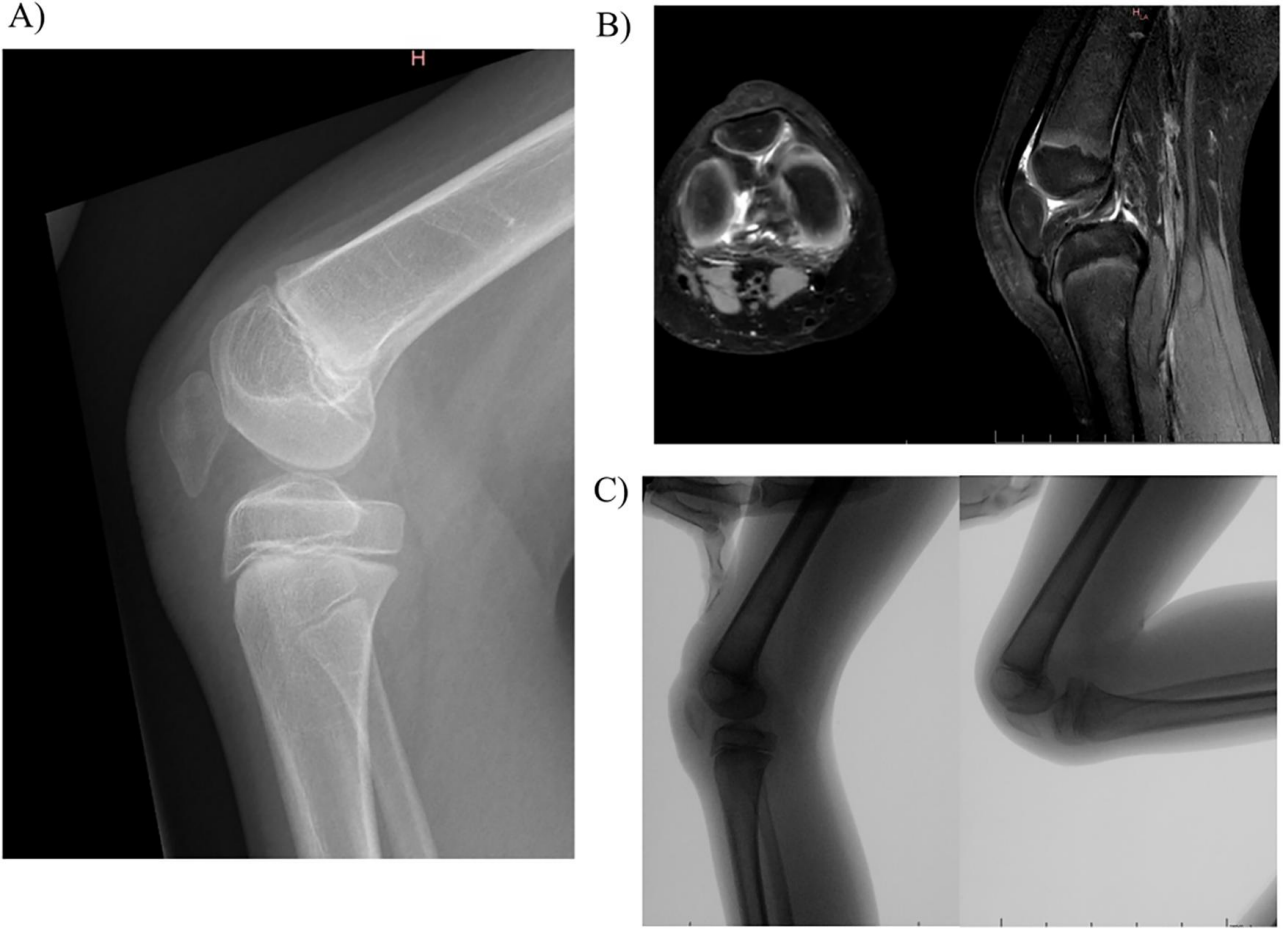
Baseline evaluation of the knee: (A) conventional radiography; (B) magnetic resonance imaging; (C) dynamic radiological examination.
